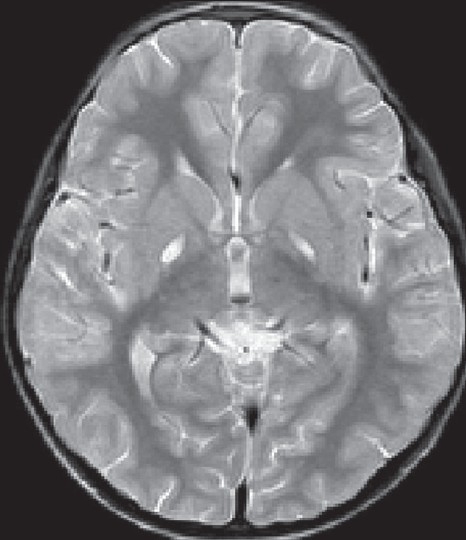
MRI (T2W) in a 12- year old boy with methylmalonic acidemia. Note the bilateral symmetrical hyperintensities involving the medial globus pallidi
